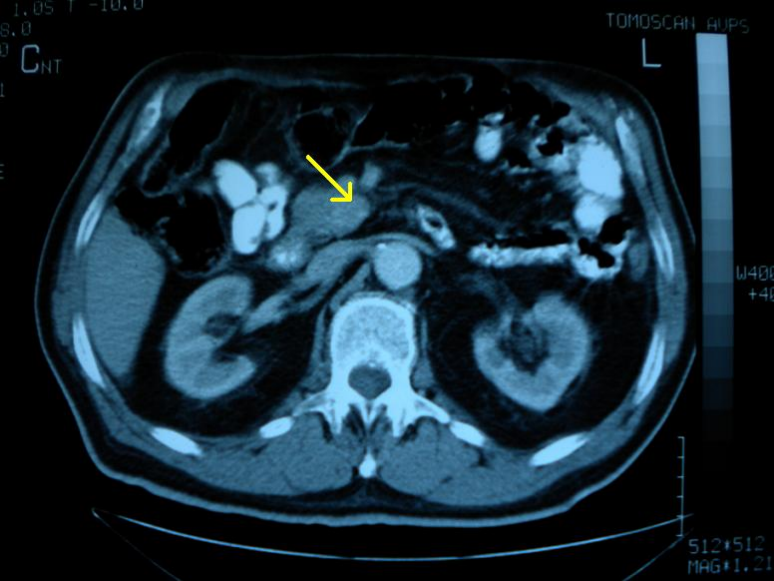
Contrast enhanced CT scan, after the first operation, demonstrating a 2 cm mass in the uncinate pancreatic process, with characteristics of an endocrine neoplasm (arrow).
